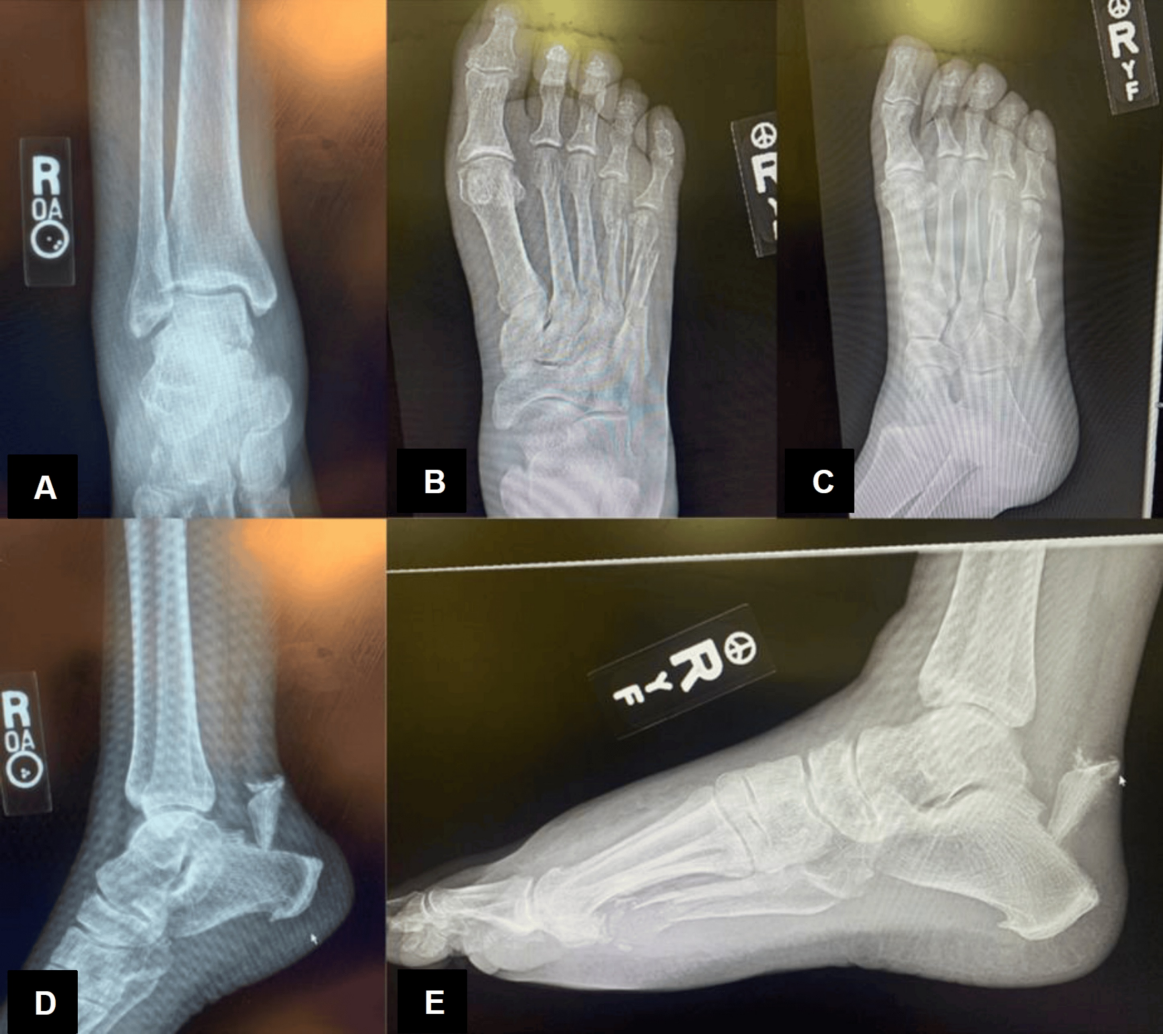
Preoperative radiographs of right foot, ankle, tibia, and fibula. Anteroposterior views of the distal tibia, fibula, ankle mortise, and foot (A and B), medial oblique of the foot (C), and lateral with and without plantar flexion (D and E), radiographs prereduction demonstrating multiple fractures of the second to the fifth metatarsals as well as a large fragment of the posterior calcaneus with 3 cm avulsion.
